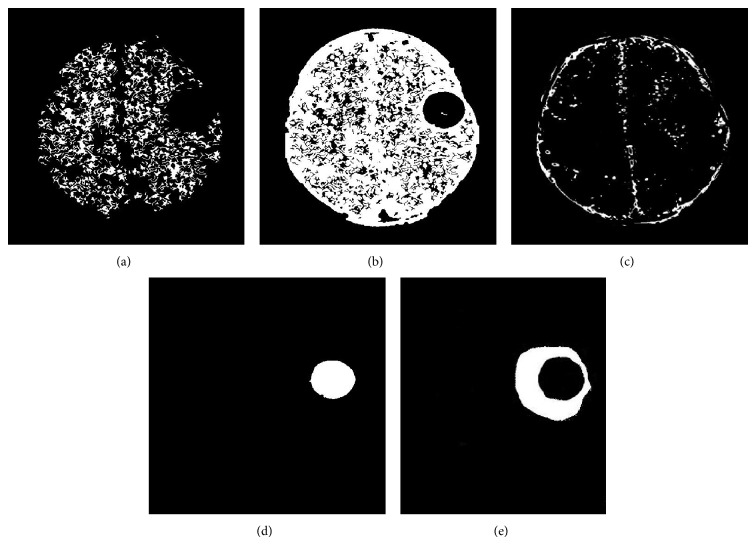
Manually segmented MRI brain tissues: (a) WM, (b) GM, (c) CSF, (d) tumor, and (e) edema.
